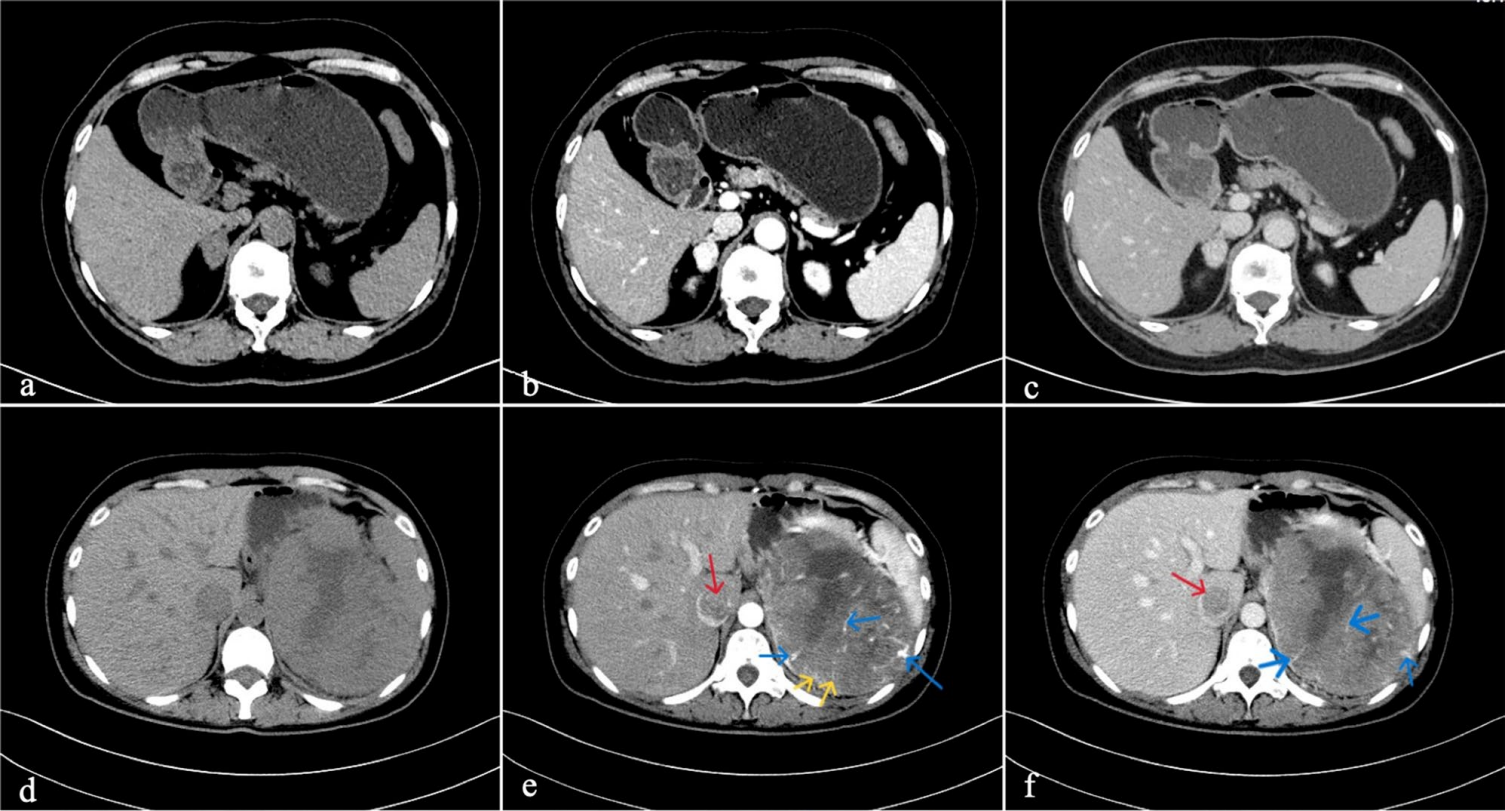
a-c. A patient with a noninvasive PCC in the right adrenal gland; the PCC had a quasicircular shape, clear boundary, and uniform density (44.8 HU) with uniform noncontrast. Obvious uneven enhancement was observed in the arterial phase, the CT value of the most obvious area of tumour solid component enhancement was approximately 168.0 HU, and the CT value of the venous phase decreased to 135.3 HU. Figure 1d and f. A patient with an invasive PCC in the left adrenal gland the PCC had a lobulated shape, unclear boundaries, and uneven density. Moreover, the streak sign (yellow arrow) and tumour vascular shadow (blue arrow) were observed in the posterior arterial phase of enhancement, and thrombus can be seen in the inferior vena cava (red arrow), and pathology suggested that the lesion had invaded the capsule
